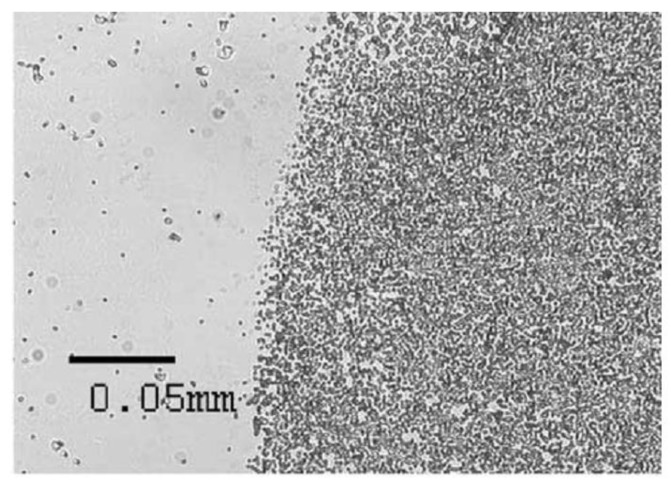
Edge of the ultrasound field that Hawkes *et al.* [51] used to deposit bacteria spores on a functionalized surface. A clear distinction between the deposition area where the field is active (right) and the inactive region (left) can be seen. Reproduced with permission from [51].
